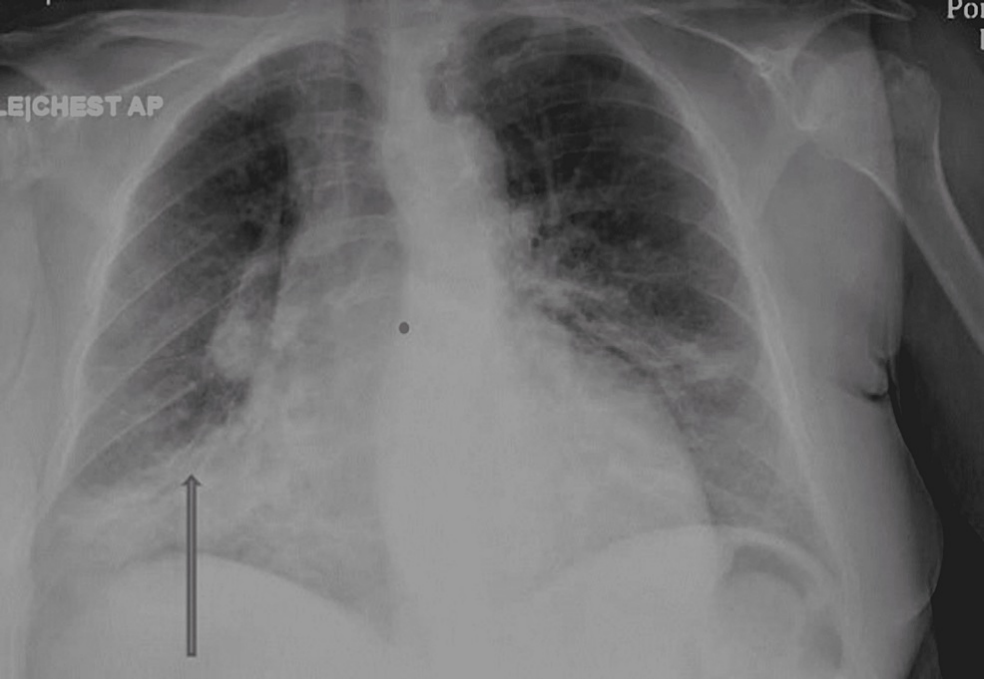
Portable chest X-ray anteroposterior view showing perihilar and bilateral lower infiltrates more on the right zone (arrow).
